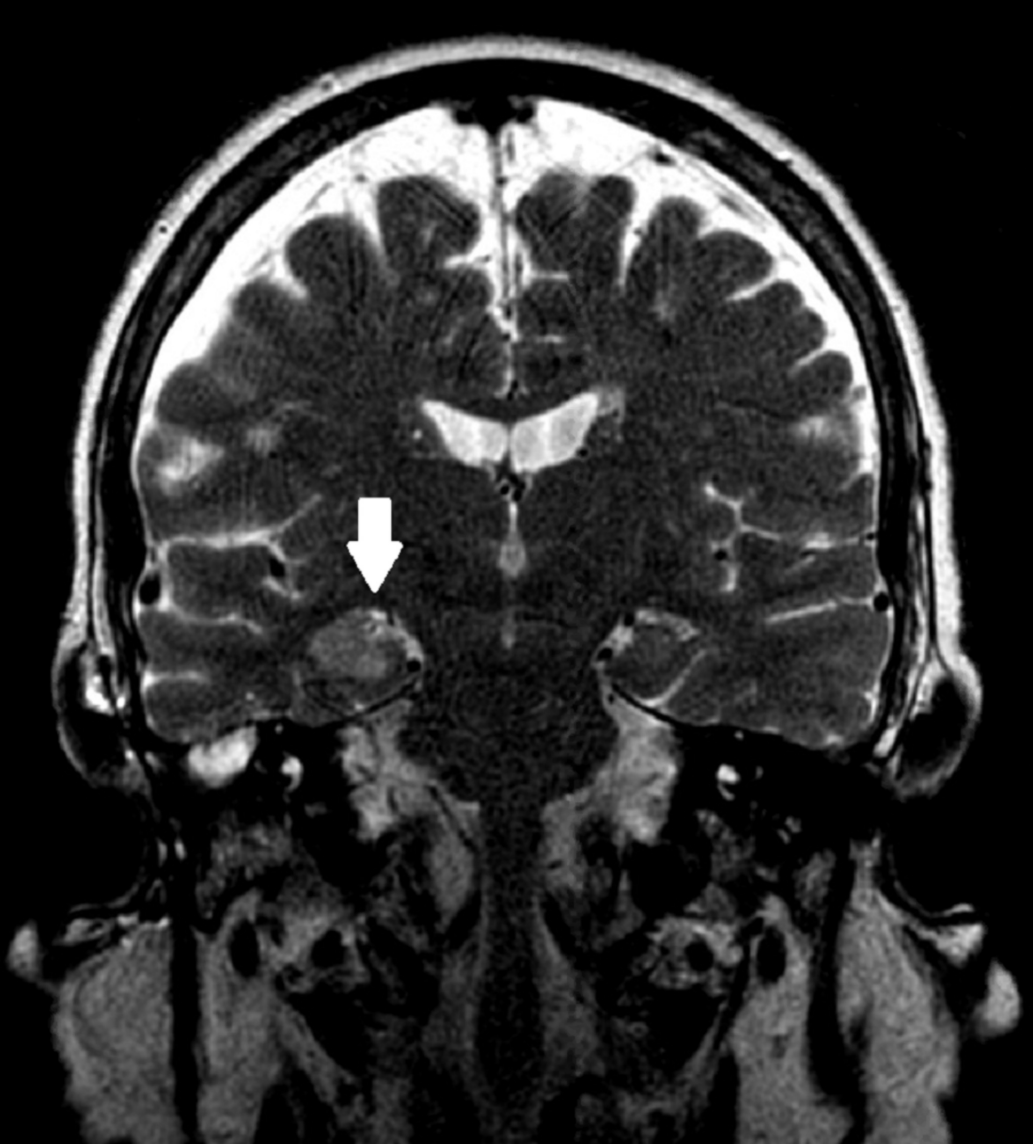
1.5 Tesla MRI protocol for epilepsy on initial presentation T2-weighted image: Arrow points to increased edema and flair within the right mesial temporal lobe MRI: magnetic resonance imaging
